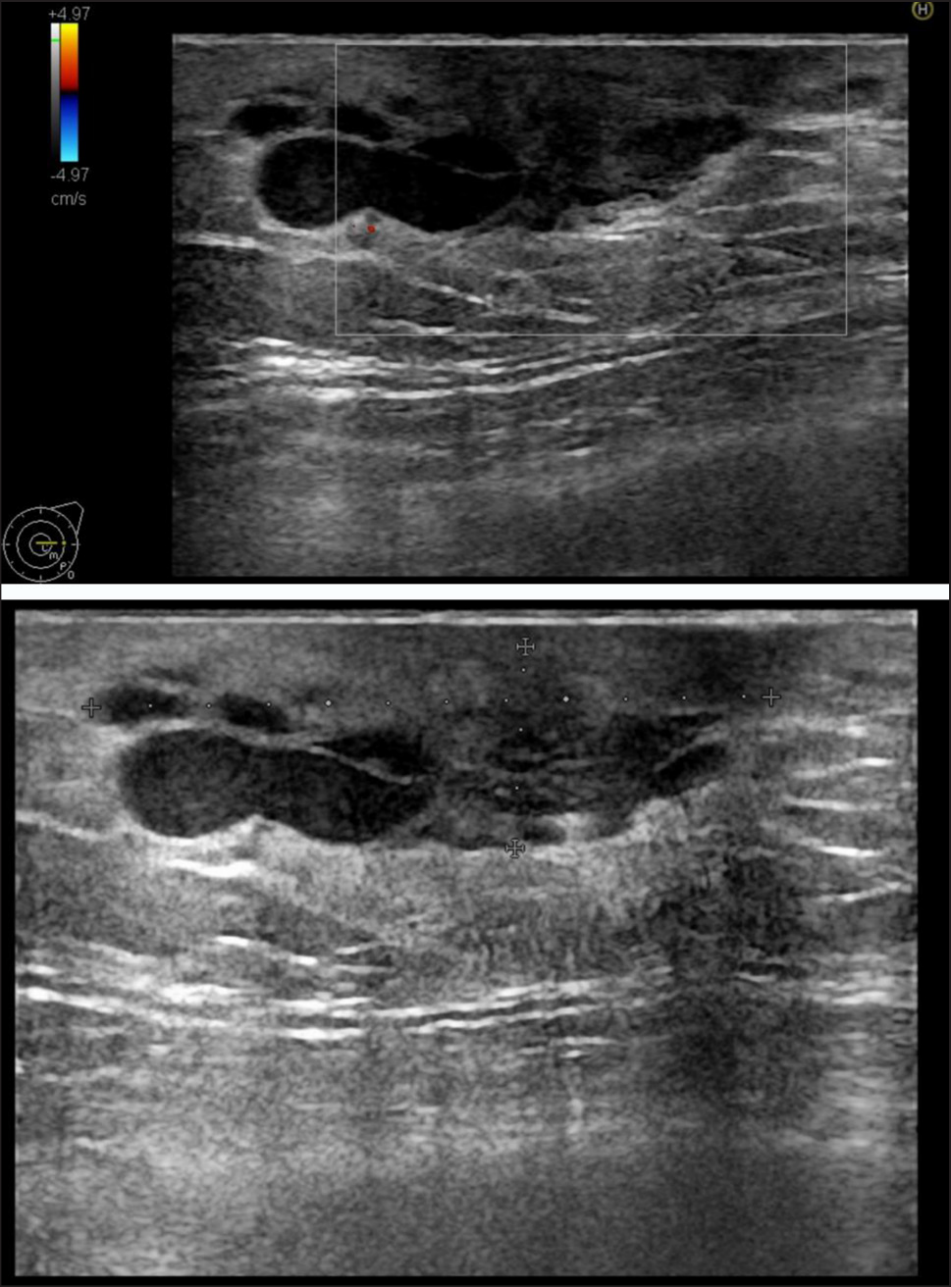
Retro-areolar hypo-echogenic mass with secondary mammary duct ectasia on ultrasound.
